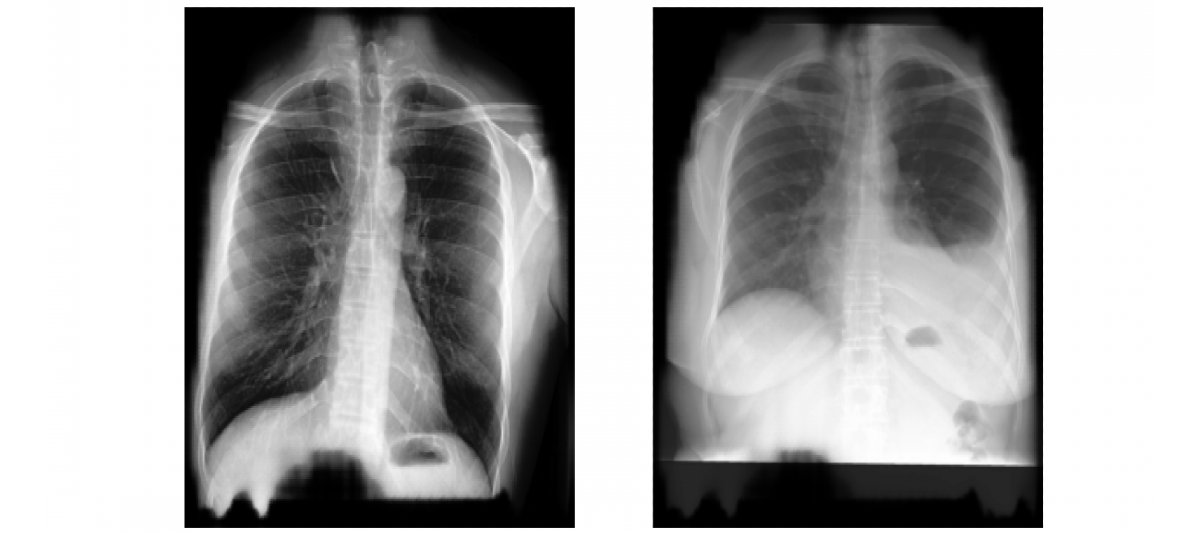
Sample segmented chest x-ray images.
